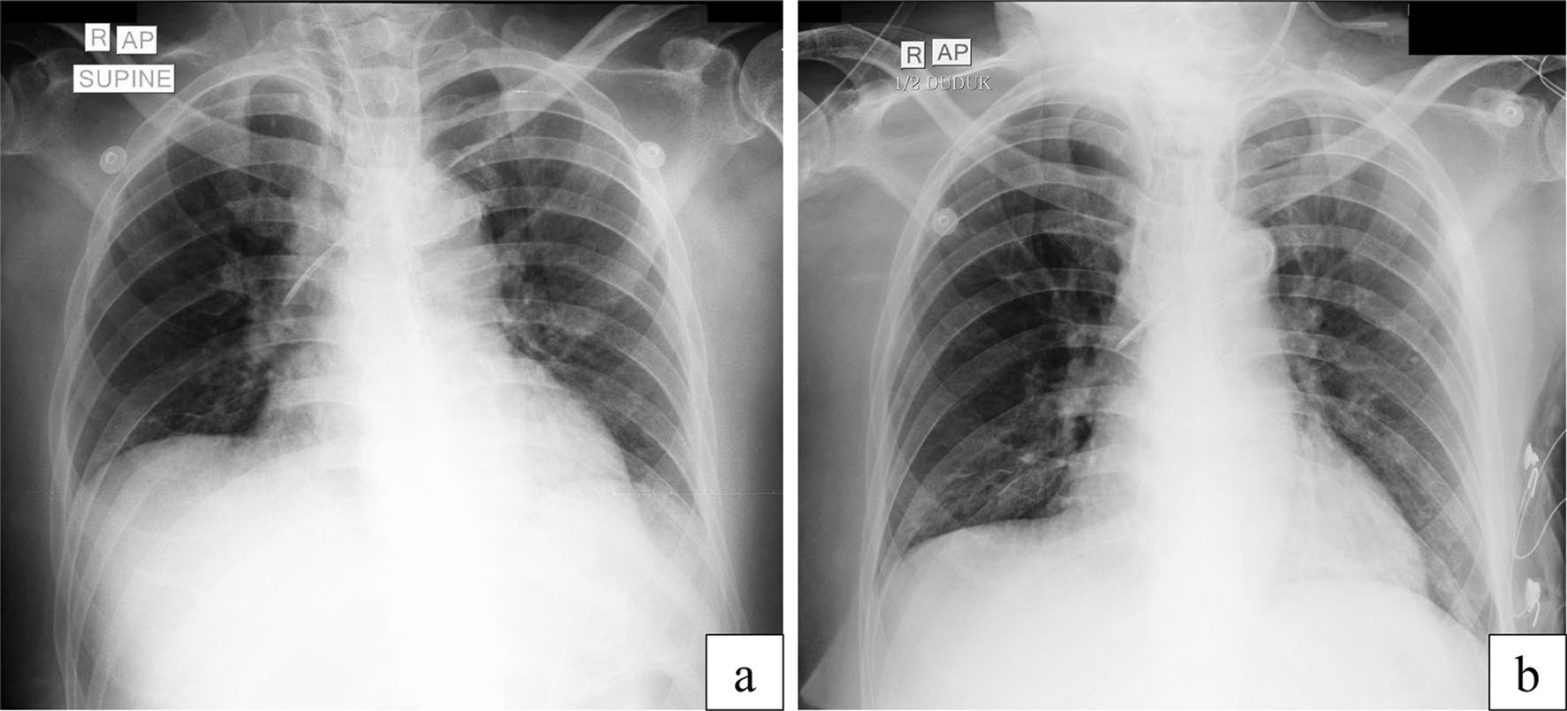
Patient’s chest x-ray when admitted to the emergency department (a), and after hemodialysis and hemoperfusion (b)
